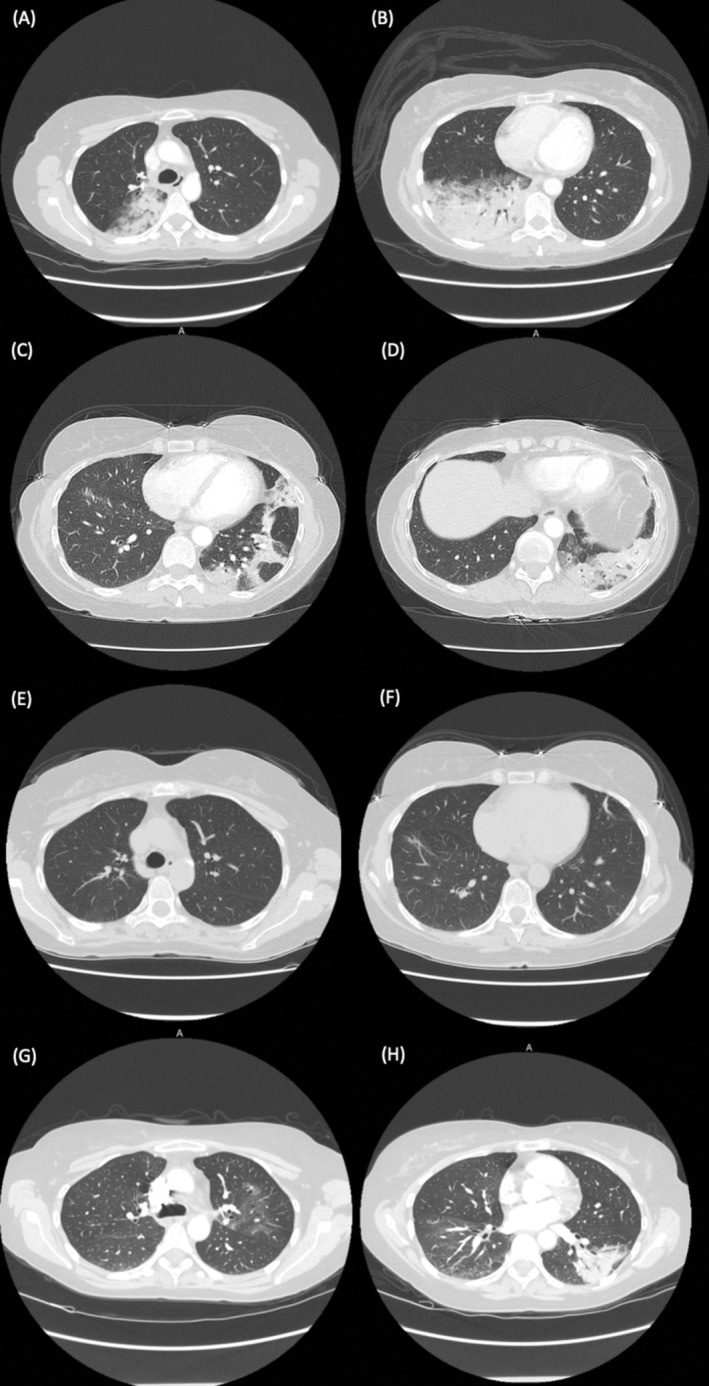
(A, B). Chest computed tomography (CT) scan axial cuts on initial admission, showing consolidation affecting the right upper and lower lobes. (C, D). Recurrence after 3‐month prednisolone taper demonstrated by consolidation in the left lower lobe and lingula, with associated ground‐glass opacities. (E, F). Complete resolution of consolidation following a 7‐month prednisolone taper. (G, H). Recrudescence of pneumonitis 2 years later, with consolidation in the left lower lobe and ground‐glass changes in the left upper lobe, lingula, and right lower lobe.
